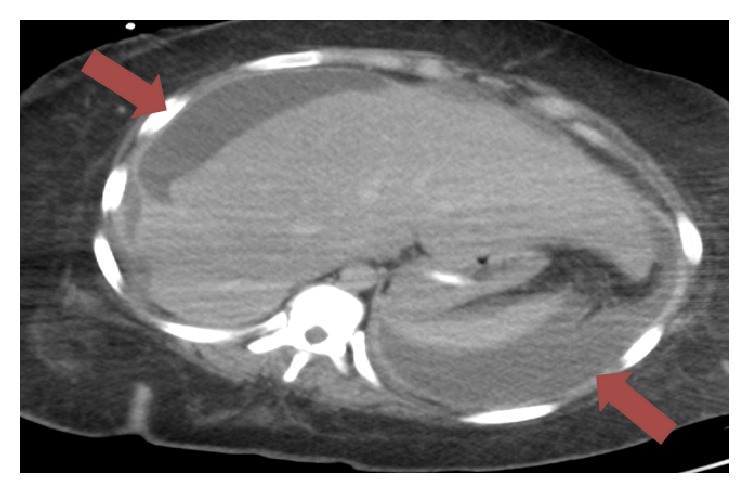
Patient's computed tomography (CT) scan of the abdomen and pelvis after her two surgeries looking for collections that remained. Red arrows demonstrate complex perihepatic and perisplenic ascites with peritoneal enhancement concerning for postoperative purulent collections. The collections were subsequently drained percutaneously and found to represent abscesses.
